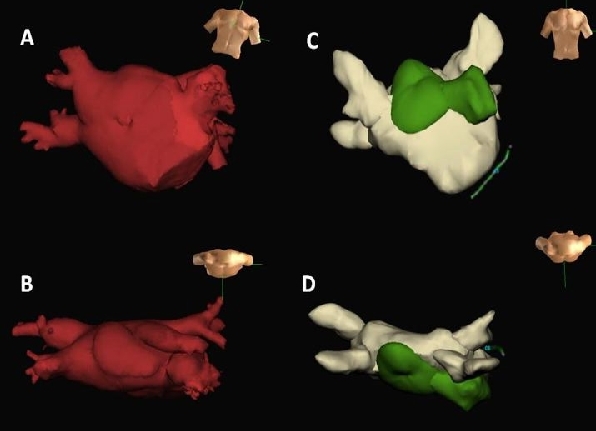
CT of left atrium in antero-posterior view (A) and viewed from above (B). Note the bulging anterior to the left sided veins which represents the anterior chamber. Panels C and D show the Ensite NavX geometry viewed in the same orientations. The infero-anterior chamber is shown in green and contains the left atrial appendage. The pulmonary veins drain into the supero-posterior chamber.
